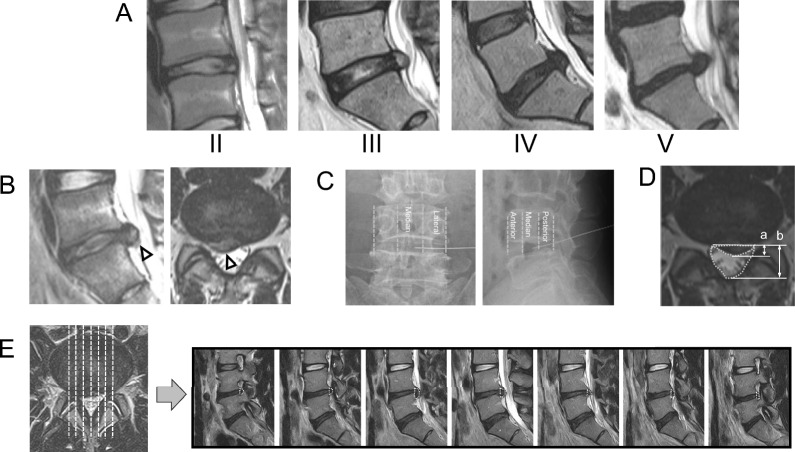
A: Degree of disc degeneration using Pfirrmann criteria. B: High intensity MRI signal in the herniation. C: Injection region. D: Bulging ratio of herniation (a/b × 100%; a: anteroposterior diameter of herniation, b: anteroposterior diameter of canal space). E: Measurement of herniated mass volume
